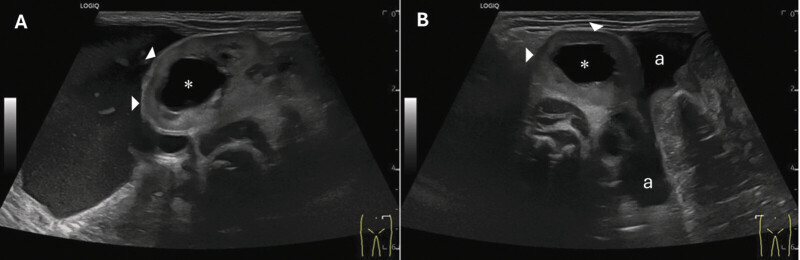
Ultrasound examination of the left (
